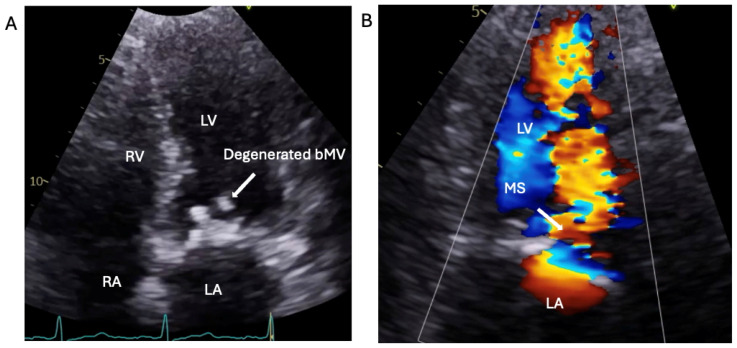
Apical 4-chamber view of transthoracic echocardiography of degenerated biological Mitral Valve (bMV), (white arrow). (A): A4CV showing calcified stenotic bMV. (B): Colour Doppler flow in diastole across degenerated bMV. RV: right ventricle; LV: left ventricle; RA: right atrium; LA: left atrium.
